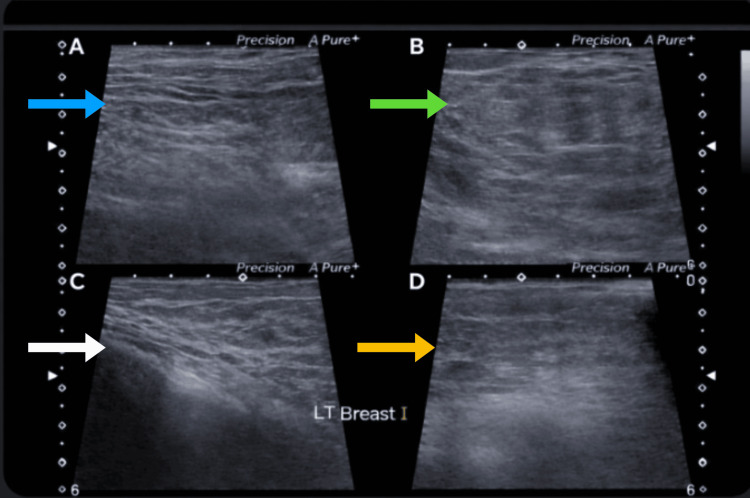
Ultrasonogram of the left breast showing the upper inner quadrant (A, blue arrow), upper outer quadrant (B, green arrow), lower inner quadrant (C, white arrow), and lower outer quadrant (D, yellow arrow), demonstrating predominantly heterogeneous tissue composition with no solid or cystic lesions, a normal nipple-areolar complex, no ductal dilatation, and a normal retroareolar region
